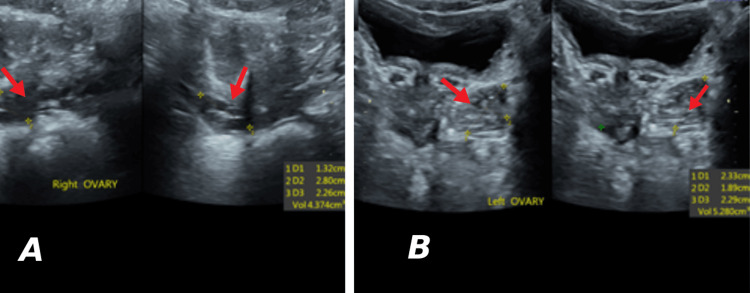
Ultrasound images showing ovaries with small follicles. A: Right ovary measuring 4.3 cm^3^; B: Left ovary measuring 5.2 cm^3^
